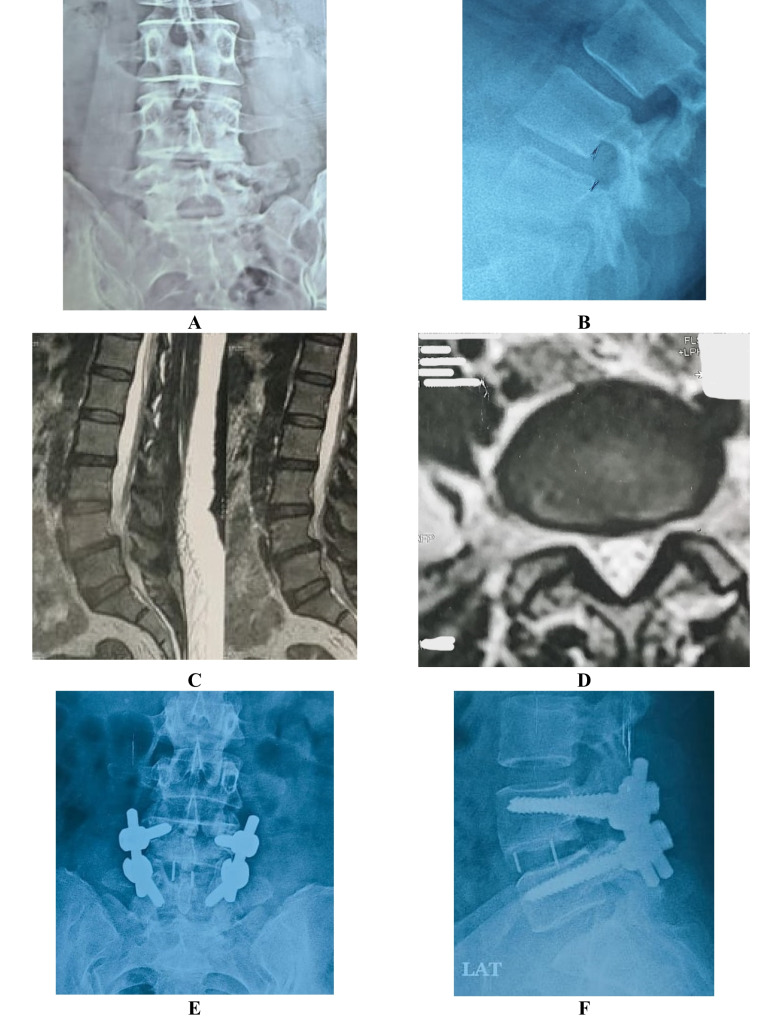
Mini-open TLIF case: (A–B) antero-posterior and lateral radiology of the slipped level and (C-D) show the sagittal and axial cut of MRI photo of that level (E–F) postoperative X-ray intro-posterior and lateral view of the slipped level
